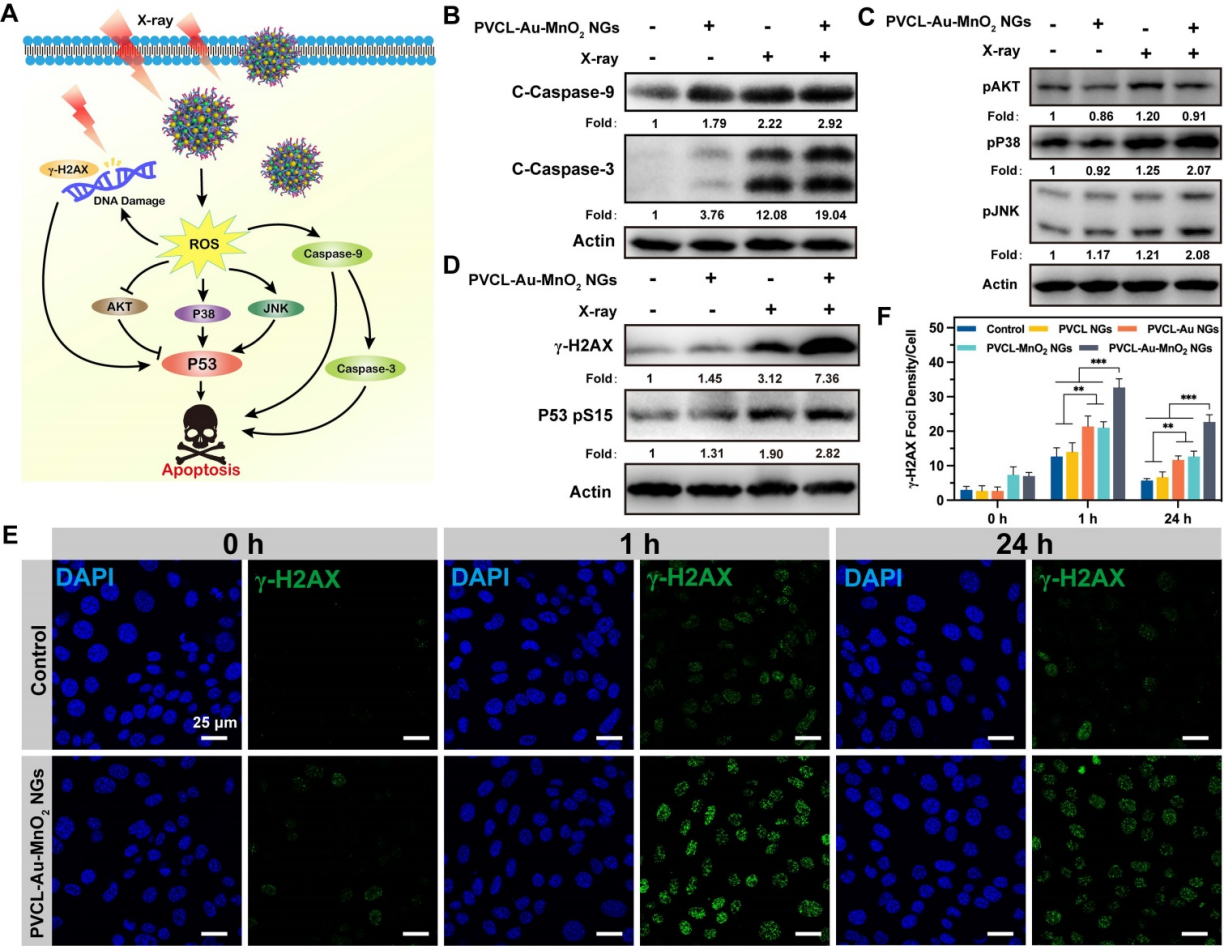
(A) Proposed molecular mechanism for the enhanced cell death triggered by PVCL-Au-MnO_2_ NGs and X-ray irradiation. Western blot analysis of the expression or phosphorylation of the (B) cleaved caspase-9/-3, (C) AKT and MAPKs signaling, and (D) γ-H2AX as well as p53 in Pan02 cells after incubation with PVCL-Au-MnO_2_ NGs (200 μg mL^-1^) with (+) and without (-) X-ray (4 Gy). (E) Change of γ-H2AX foci (green) in cell nuclei (blue) of Pan02 cells treated with PVCL-Au-MnO_2_ NGs plus X-ray (4 Gy) or by X-ray alone (control). (F) The corresponding γ-H2AX foci density in Pan02 cells at different time points post X-ray irradiation (n = 5, * for *p* < 0.001 and  for *p* < 0.01, respectively).
